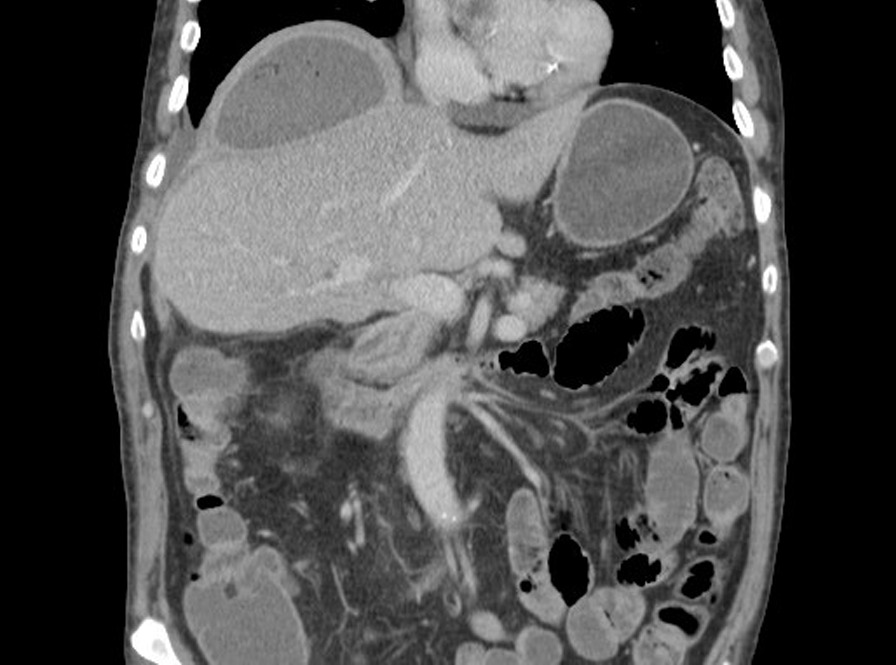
Coronal CT image in a patient with fever and abdominal pain one week after cholecystectomy. There is elevation of the right hemidiaphragm with an encapsulated collection containing fluid and gas between the diaphragm and the surface of the liver consistent with subphrenic abscess. Case coutesy of Dr Vitalii Rogalskyi, Radiopaedia.org, rID: 66300
